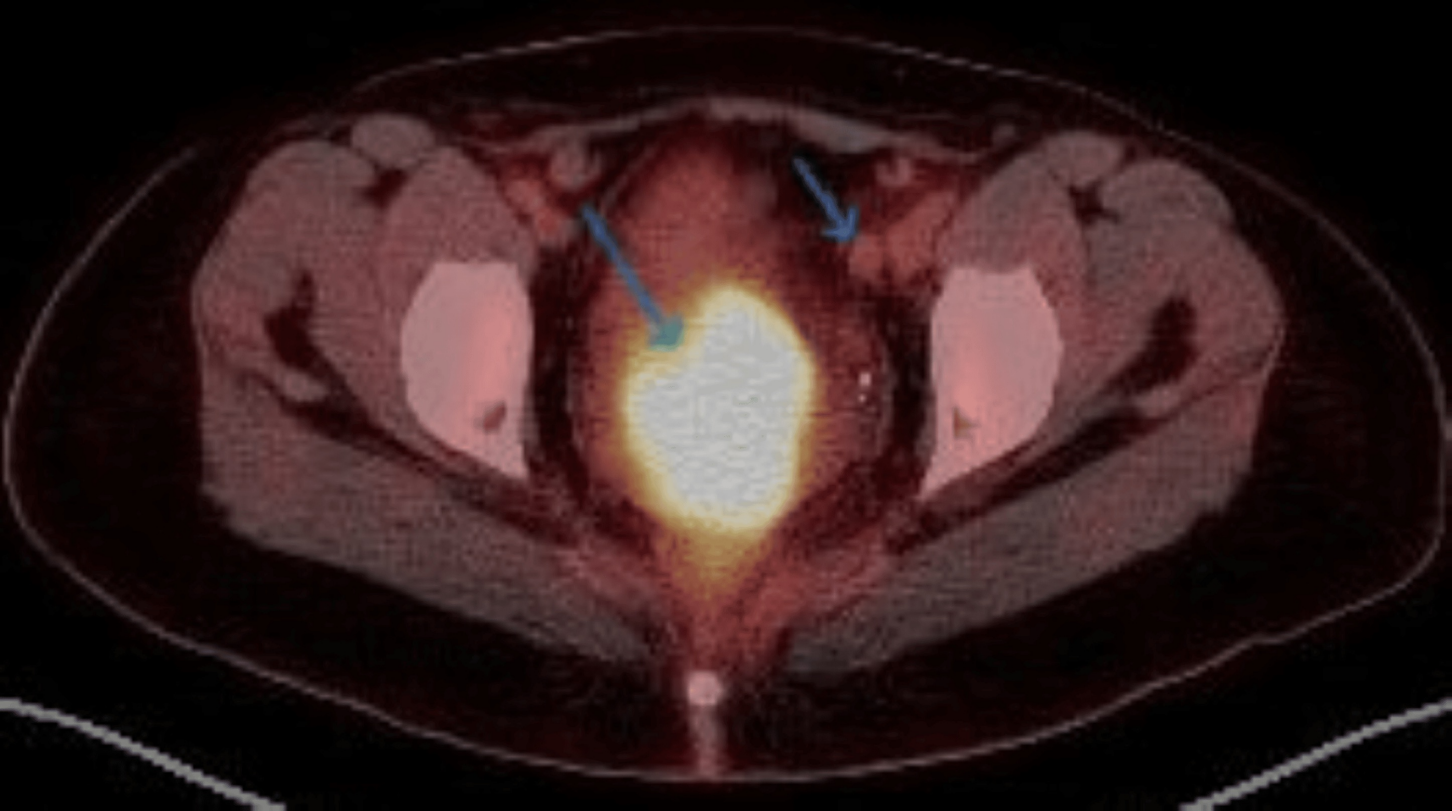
FDG PET-CT demonstrates a strongly avid cervical mass and a mildly avid left external iliac lymph node. FGD: fluorodeoxyglucose; PET-CT: positron emission tomography-computed tomography
